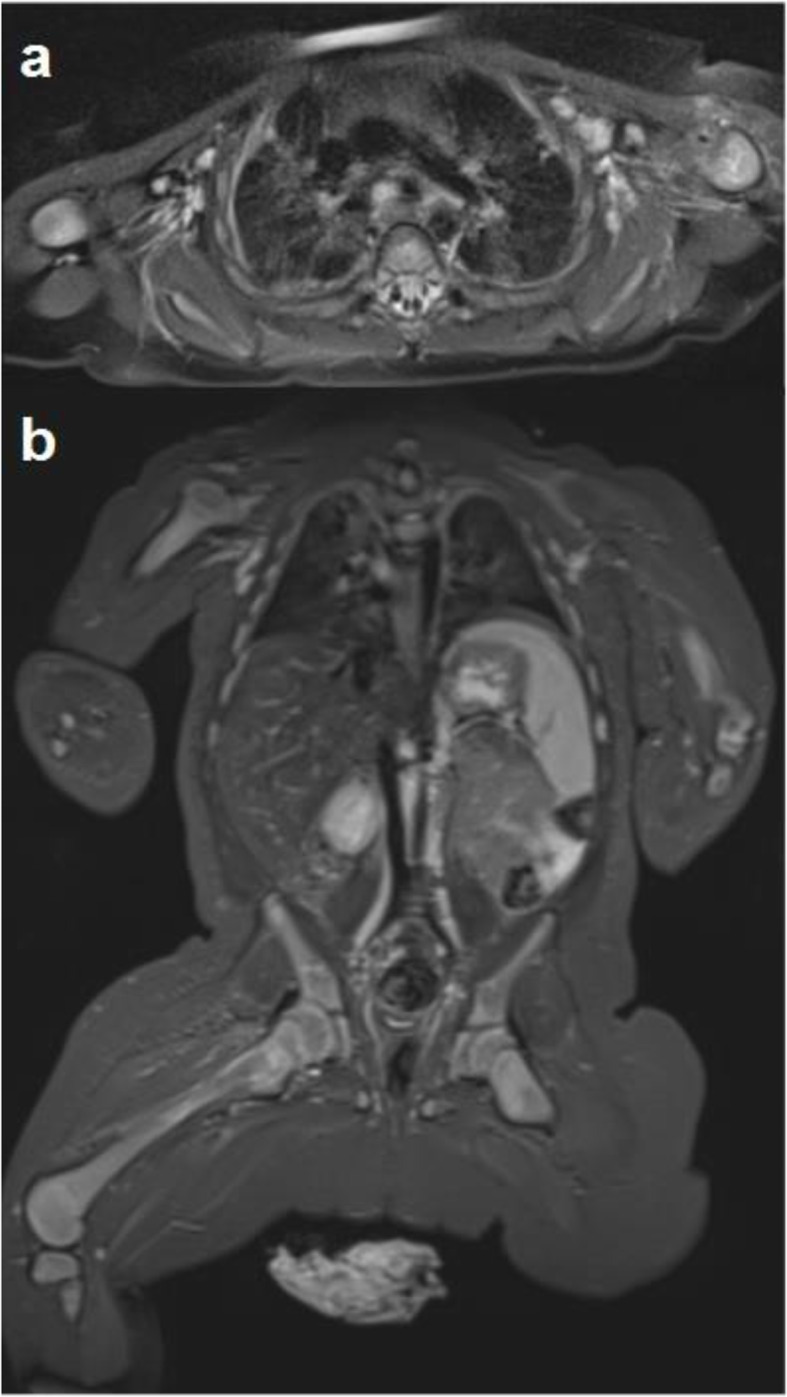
a-axial and b-coronal STIR MR images performed about 1 month later showed complete resolution of muscular involvement and improvement of lungs and bone flogistic involvement
